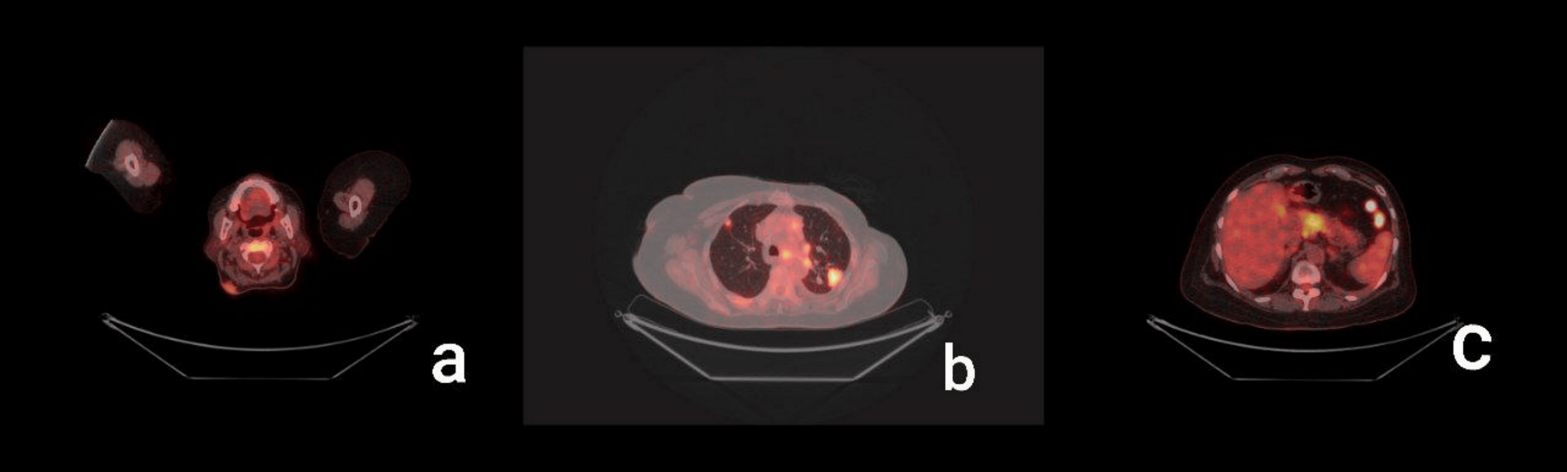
PET scan images showing FDG-avid uptake in the (a) neck, (b) lung, and (c) pancreas, suggestive of metastasis.
